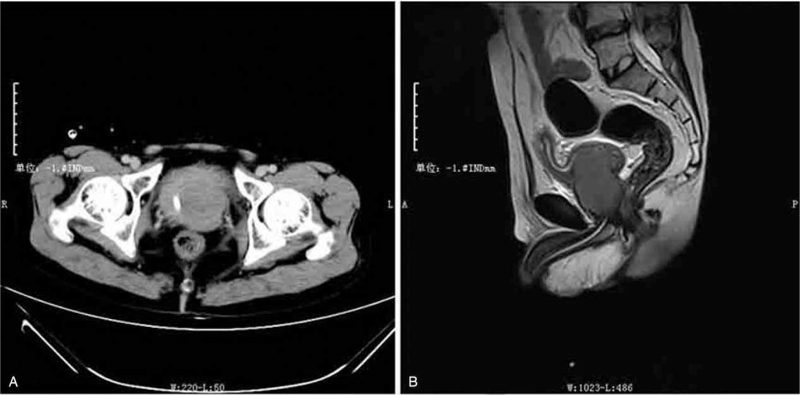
CT imaging (A) and T2 weighted MRI (B) revealed a 5.0 × 4.0 cm neoplasm of the left lobe of the prostate with invasion of the bladder neck and left seminal vesicle.
